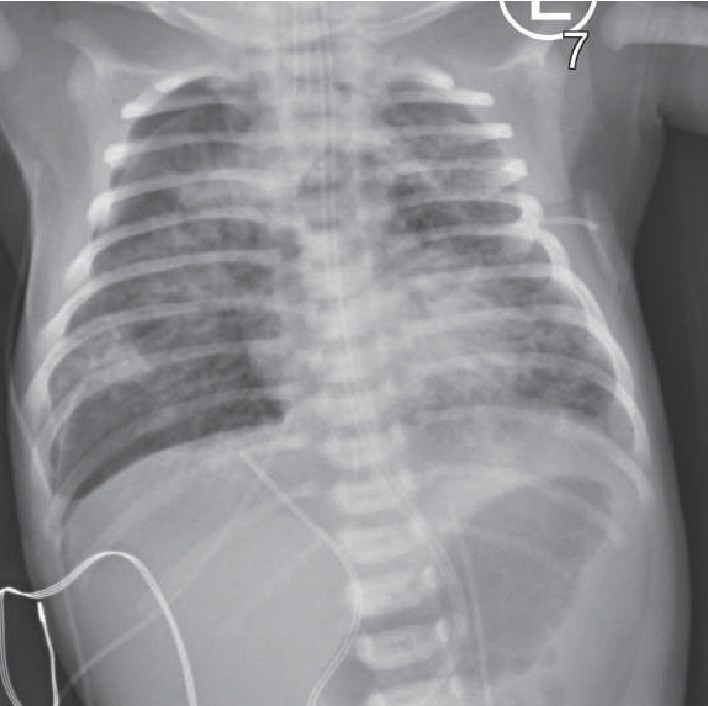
Chest radiograph revealing moderately sized right-sided pneumothorax and pneumomediastinum. Diffuse coarse bilateral pulmonary opacities consistent with MAS.
